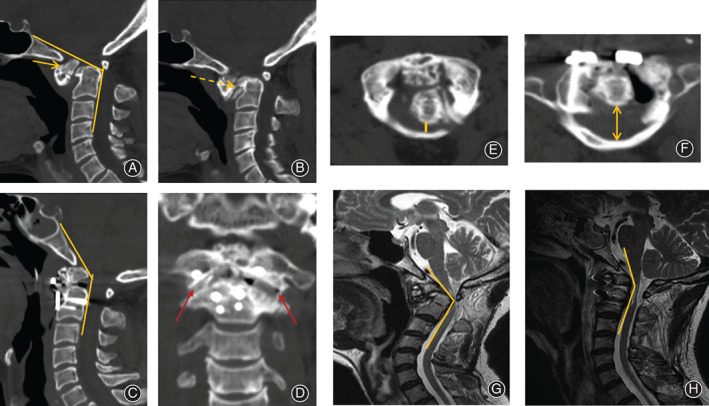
Comparison of CT and MRI preoperatively and postoperatively. (A, C) The clivus‐canal angle (CCA) was significant improved from 107.15° preoperatively to 138.37° postoperatively. Os odontoideum (yellow arrow) was fused with the anterior arch of the atlas. (B) Preoperative CT demonstrated that there was bone connection (yellow dotted line arrow) between the odontoid vertebra and the atlas, and the end of the dens was smooth. (D) Coronal plane CT shows the bone graft (red arrow) in lateral mass joint of bilateral atlantoaxial spine. (E, F) Significant widening of the posterior atlantodental interval from 2.94 mm preoperatively to 15.41 mm postoperatively. (G) Ventral spinal cord compression and myelomalacia could be found in preoperative MRI. (H) Significant improvement of the cervicomedullary angle (CMA) from 118.64° preoperatively to 147.32° postoperatively.
